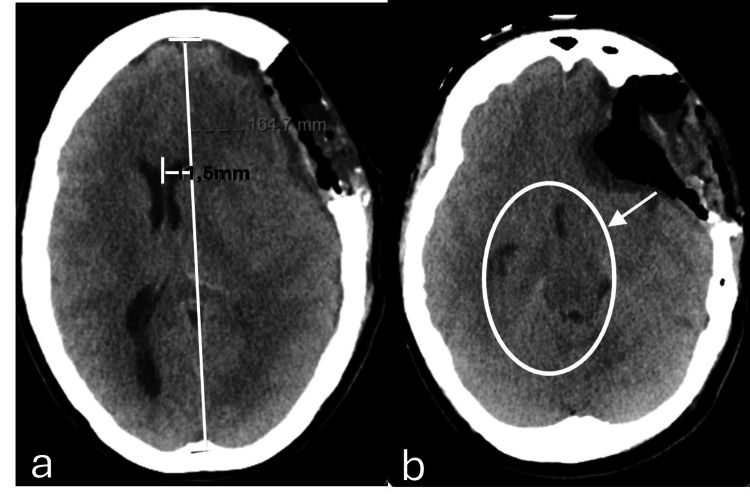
Axial plane of brain computed tomography after decompressive craniectomy (a) Mass effect remains on the left cerebral hemisphere and on the ventricular system, with signs of subfalcine hernia and clear deviation of the midline structures to the right, approximately 11.5 mm (the previous examination was approximately 10.7 mm). (b) An outline of an uncal hernia (white arrow), with molding of the midbrain and decreased permeability of the base cisterns (white circle).
